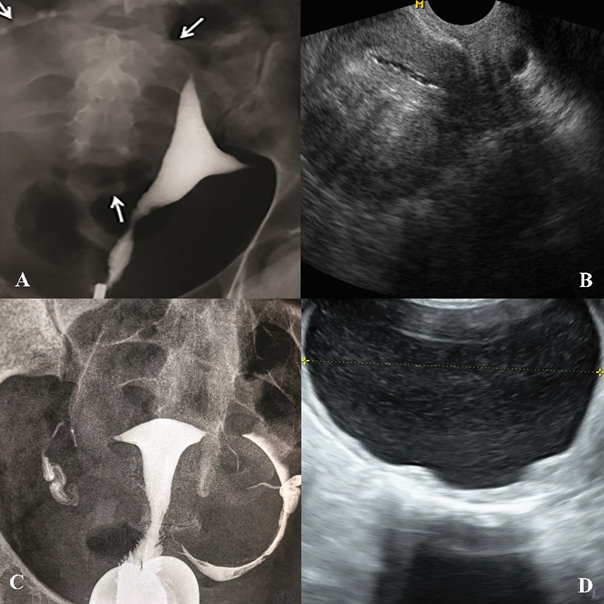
A) HSG of a 27-yr-old patient with primary infertility. A large soft tissue mass causing the elongation of the right wall and dislocation of the uterus to the left. B) An ultrasound image of patient “A”, which confirms the presence of a large fibroid. C) Dislocation of the left fallopian tube due to a soft tissue mass in HSG of a 37-yr-old patient. D) Ultrasound image of patient “C”, that shows an ovarian endometrioma cyst.
